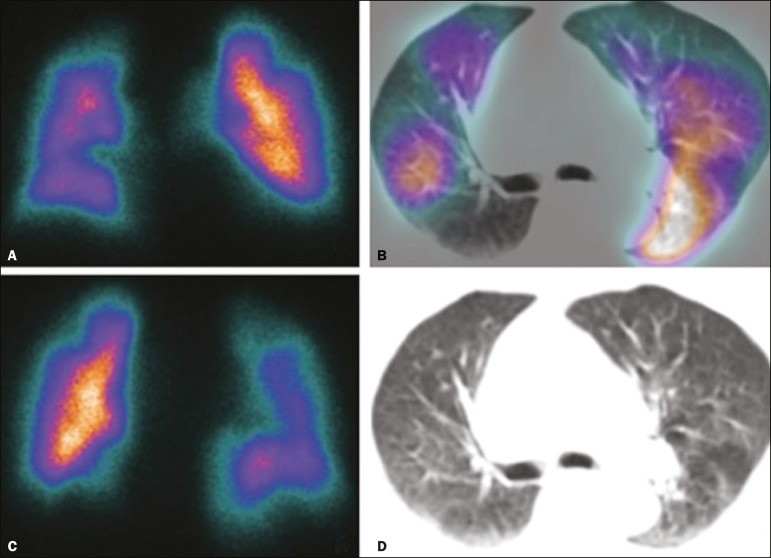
Pulmonary perfusion scintigraphy in coronal sections
